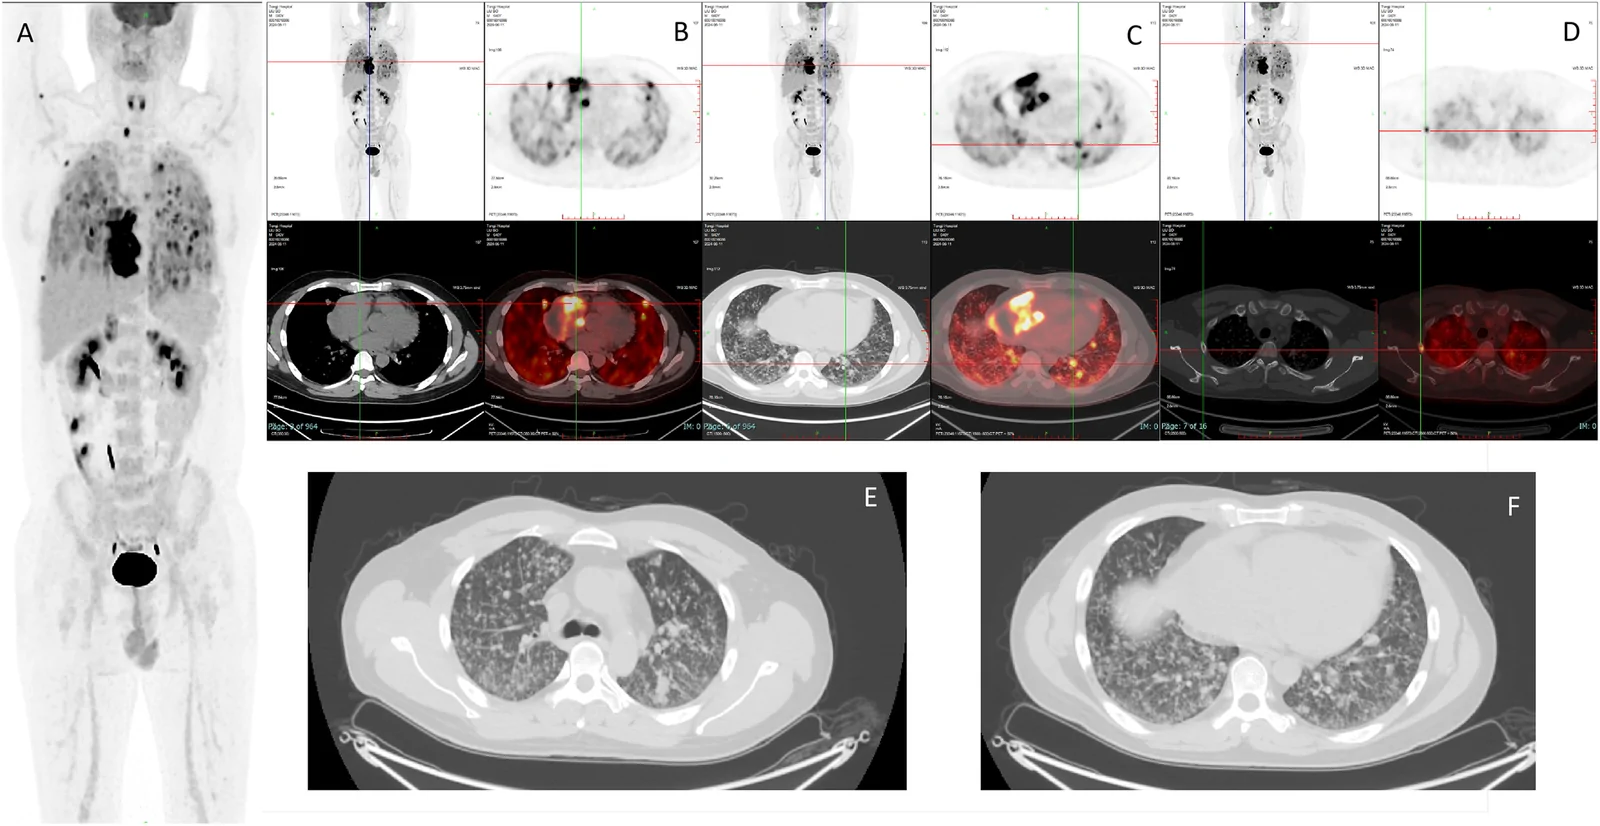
Whole-body PET/CT scan results: (A–D) reveal multiple soft-tissue masses/nodules around bilateral atria, pericardium, and mediastinum with elevated metabolism; diffuse lung nodules of varying sizes with increased metabolism; enlarged adrenal nodules with hypermetabolism; and focal metabolic upticks in the right humerus and right third/seventh ribs. (E,F) show diffuse lung nodules of different sizes.
